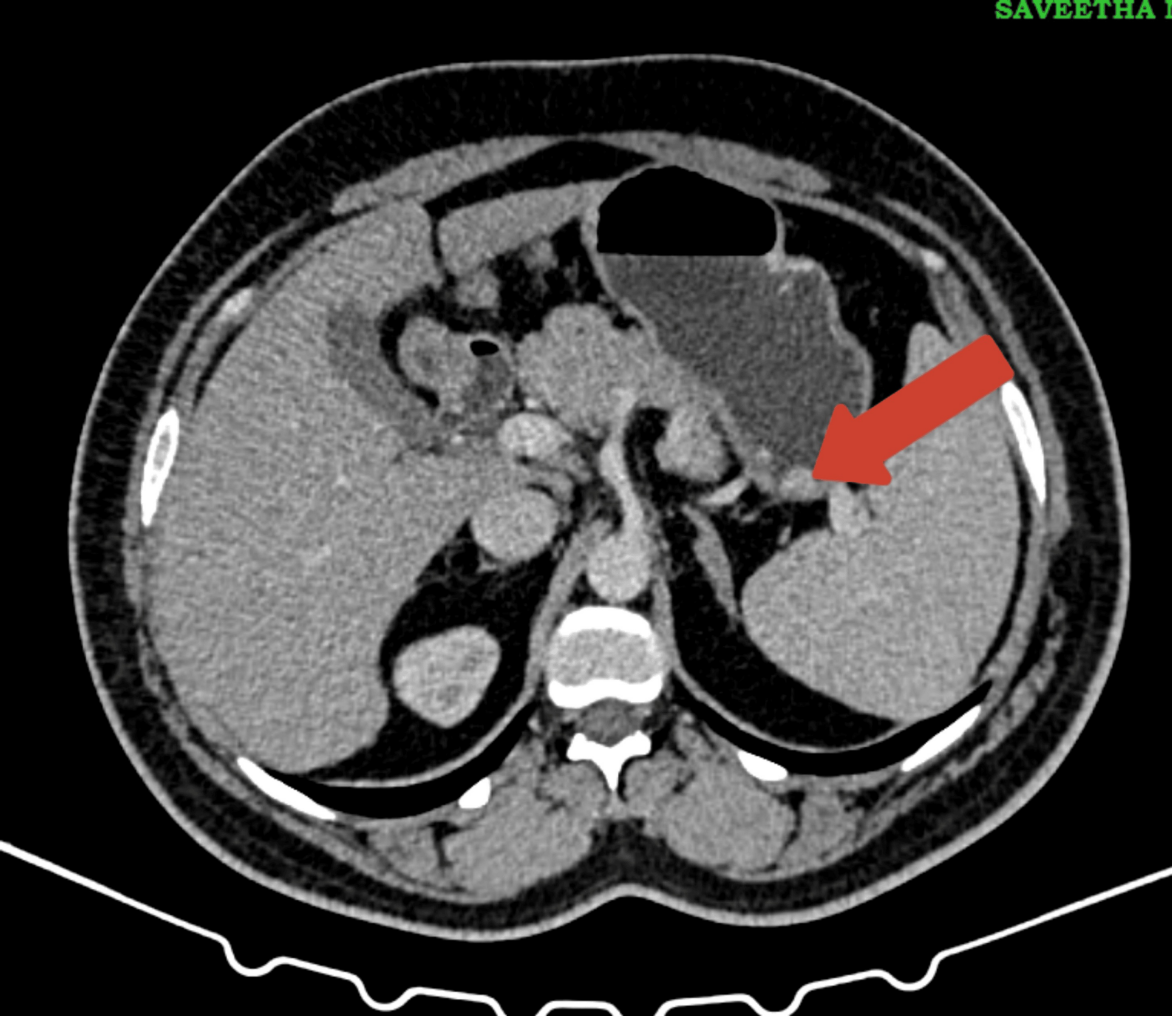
Contrast-enhanced CT (CECT) of the abdomen showing thickening in the greater curvature of the stomach. The axial CECT image of the abdomen highlights a thickened segment of the greater curvature of the stomach (indicated by the red arrow).
